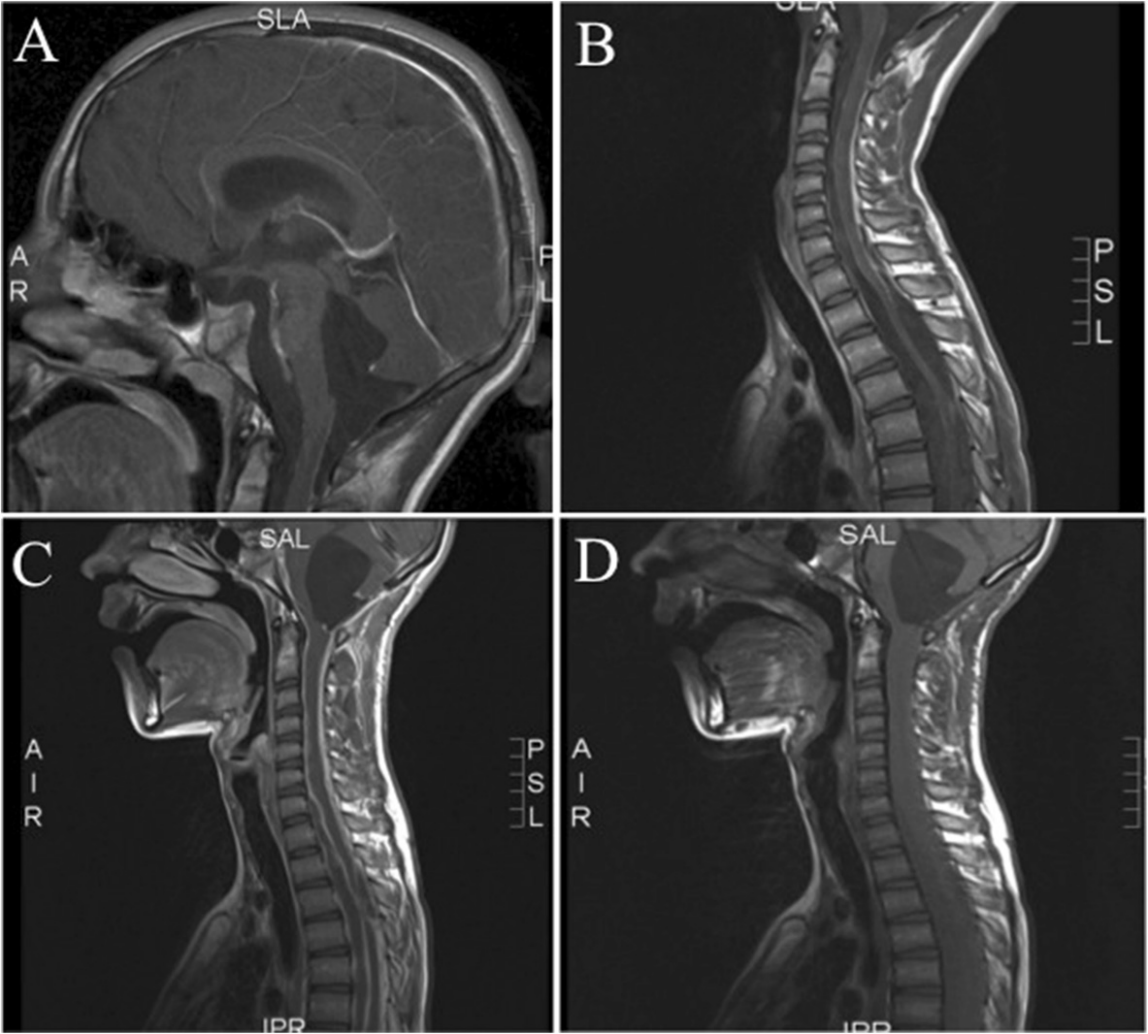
Brain and spinal MRI images. A. MRI of the brain showed an enlarged fourth ventricle in contact with a retrocerebellar cyst extending through the foramen magnum, and an enlarged subarachnoid cistern anterior to the brainstem, extending from the medulla oblongata towards the floor of the third ventricle. The brain stem appeared to be compressed or atrophied due to the combined pressure from these cysts. T1 weighted image with contrast. B. Spinal MRI showed a widened spinal canal and a distorted and atrophic spinal cord, due to the presence of multiple cysts with extensive leptomeningeal and dural contrast enhancement without the presence of solid masses. T1 weighted image with contrast. C and D. MRI of the brain and spinal cord, a few months after surgery, showed progressive leptomeningeal lesions of the brain and spinal cord with (further) compression of these structures and hydrocephalus (C with, D without contrast).
